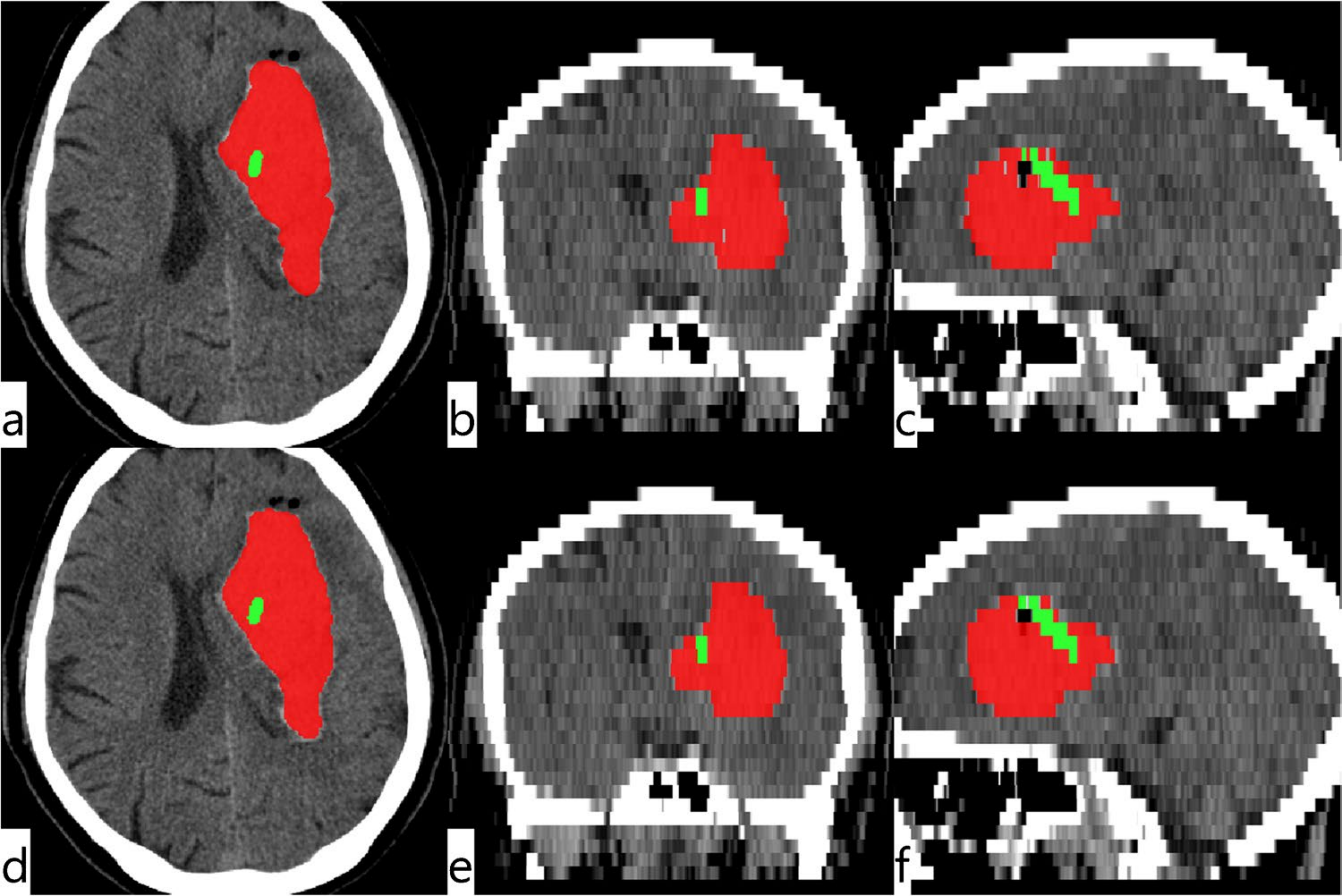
CT images of a test patient. a & d axial, b & e coronal and c & f sagittal reformats of the CT scan with GT (top row) and predicted masks (bottom row) of ICH (red) and the drain (green)
